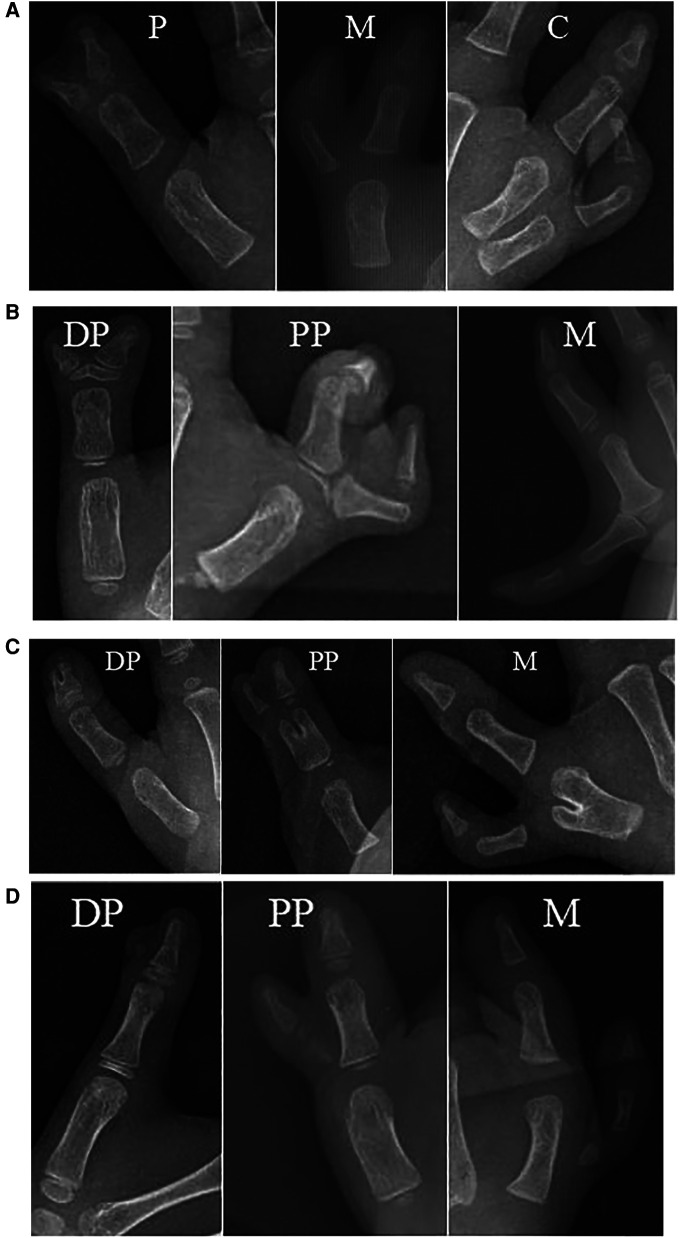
(A–D) Radiograph of the Chung et al. Classification. (A) type A-P, A-M, A-C; (B) type B-DP, B-PP and B-M; (C) type C-DP, C-PP and C-M; (D) type D-DP, D-PP and D-M. P, phalanx; M, metacarpal; C, carpal; DP, distal phalanx; PP, proximal phalanx.
